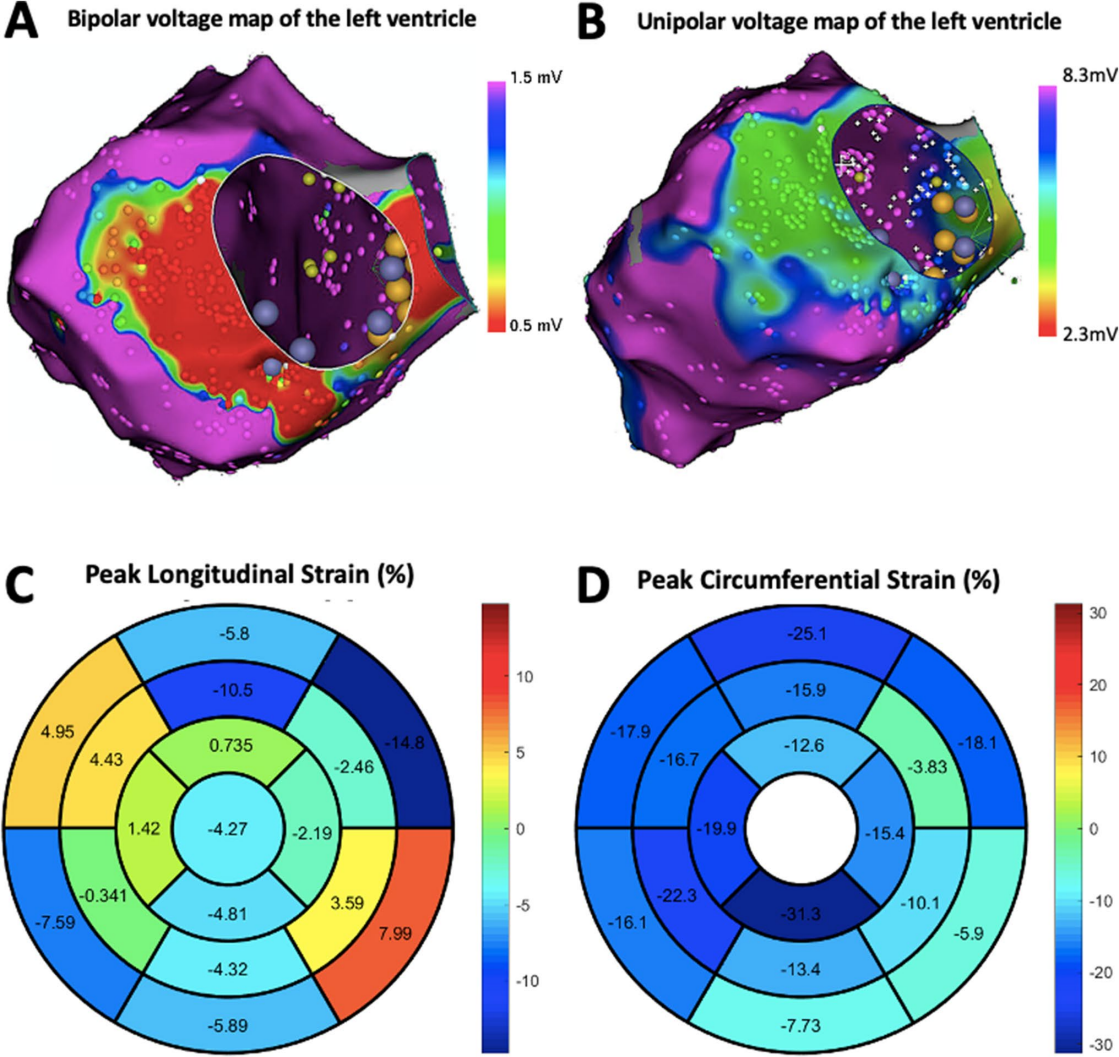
Case demonstration: Panel A shows the bipolar voltage map by electroanatomical mapping in a patient of non-ischemic cardiomyopathy with low voltage zones in the inferolateral basal left ventricle; Panel B shows the unipolar voltage map by electroanatomical mapping in the same patient with low voltage zones in the anterolateral and inferolateral basal left ventricle; Panels C and D show the polar plot of segmental distribution of peak longitudinal and circumferential strain respectively in this patient which correlates with both the bipolar and unipolar low voltage zones. Cardiac magnetic resonance imaging with late gadolinium enhancement had failed to detect any abnormality in this region
